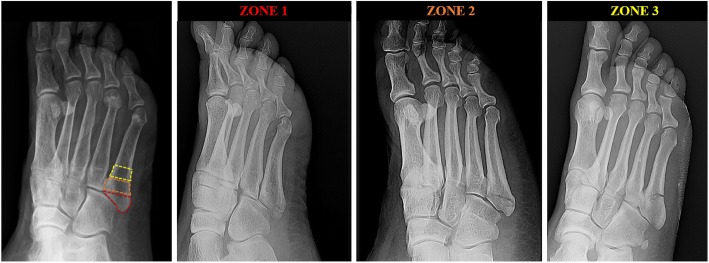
Radiographic classification of the fractures according to Lawrence and Botte^7^. The three different zones are based on anatomic landmarks (far left): proximal to the meta-diaphyseal junction involving the tuberosity (zone 1, centre left), between the lines of the intermetatarsal joint between fourth and fifth metatarsal bones involving the meta-diaphyseal junction and the intermetatarsal articular facet (zone 2, centre right), distal to the meta-diaphyseal junction involving the proximal diaphysis (zone 3, far right)
